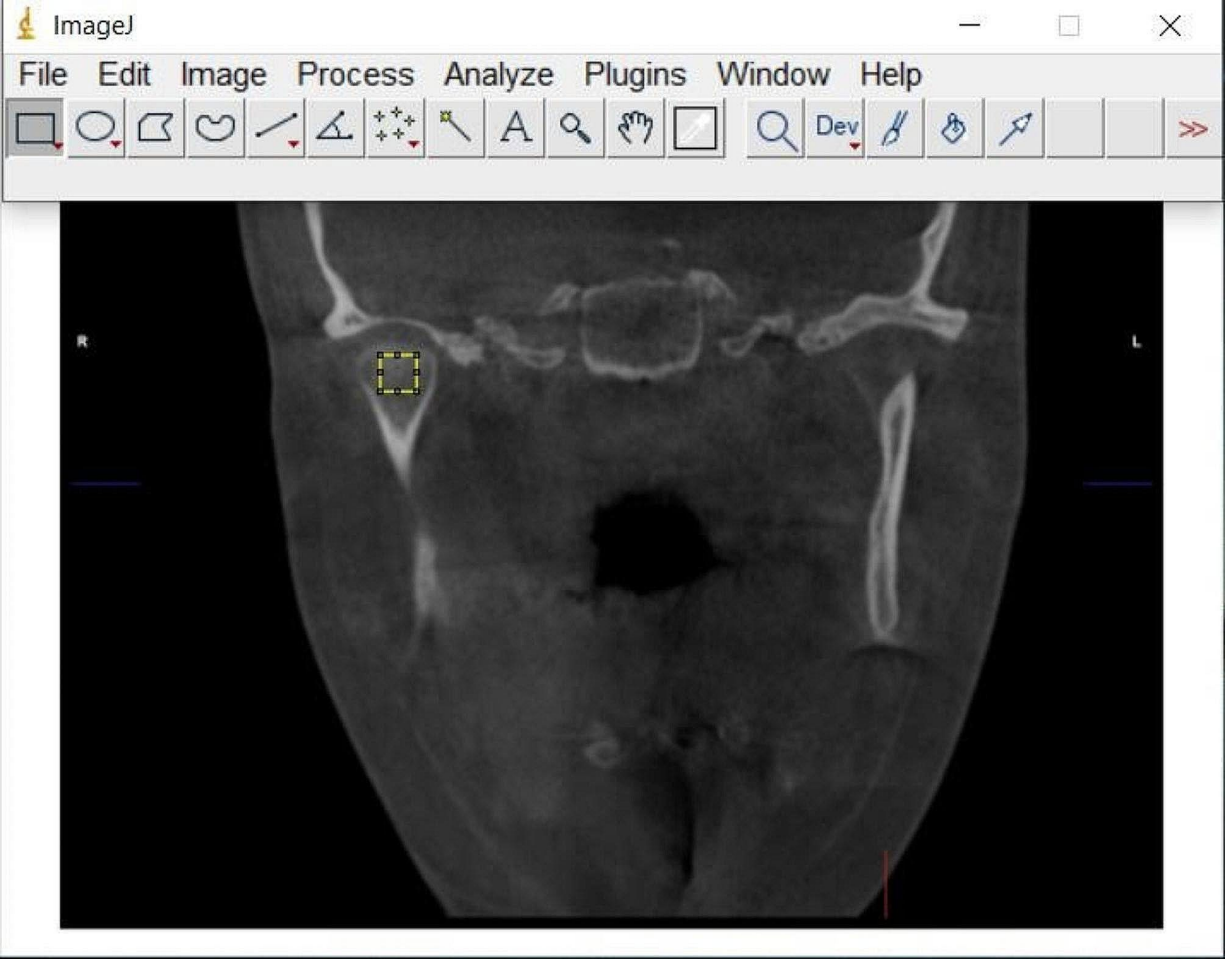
ROI selection on coronal CBCT image
